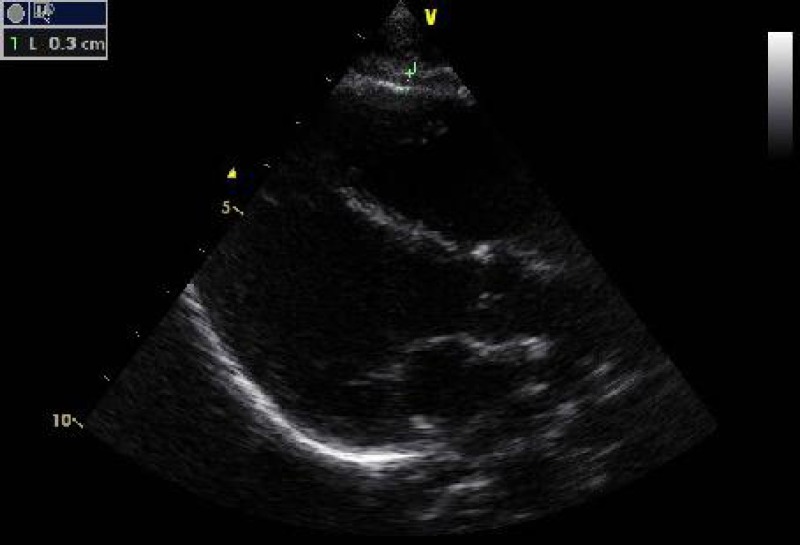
Echocardiographic measurement of epicardial adipose tissue (EAT)(plus signs indicate epicardial area)
